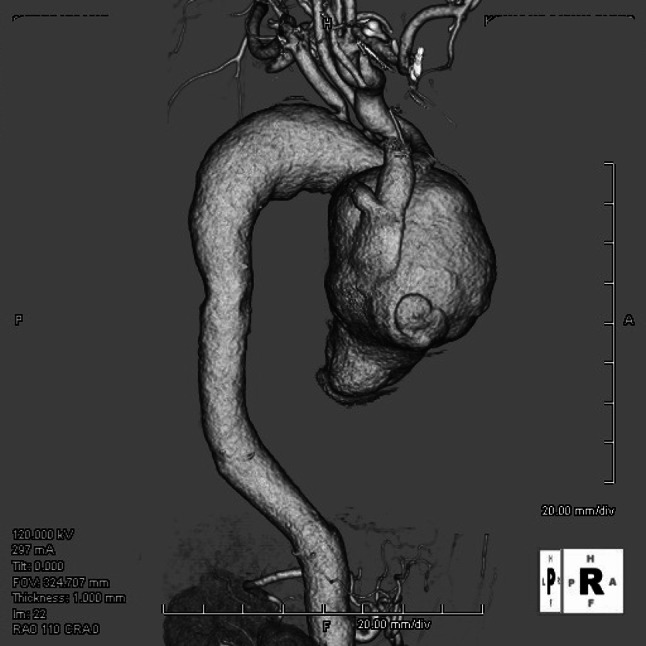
Preoperative computed tomography showing giant ascending aortic aneurysm with the dimension of 79 mm in size and severely compressed SVC
